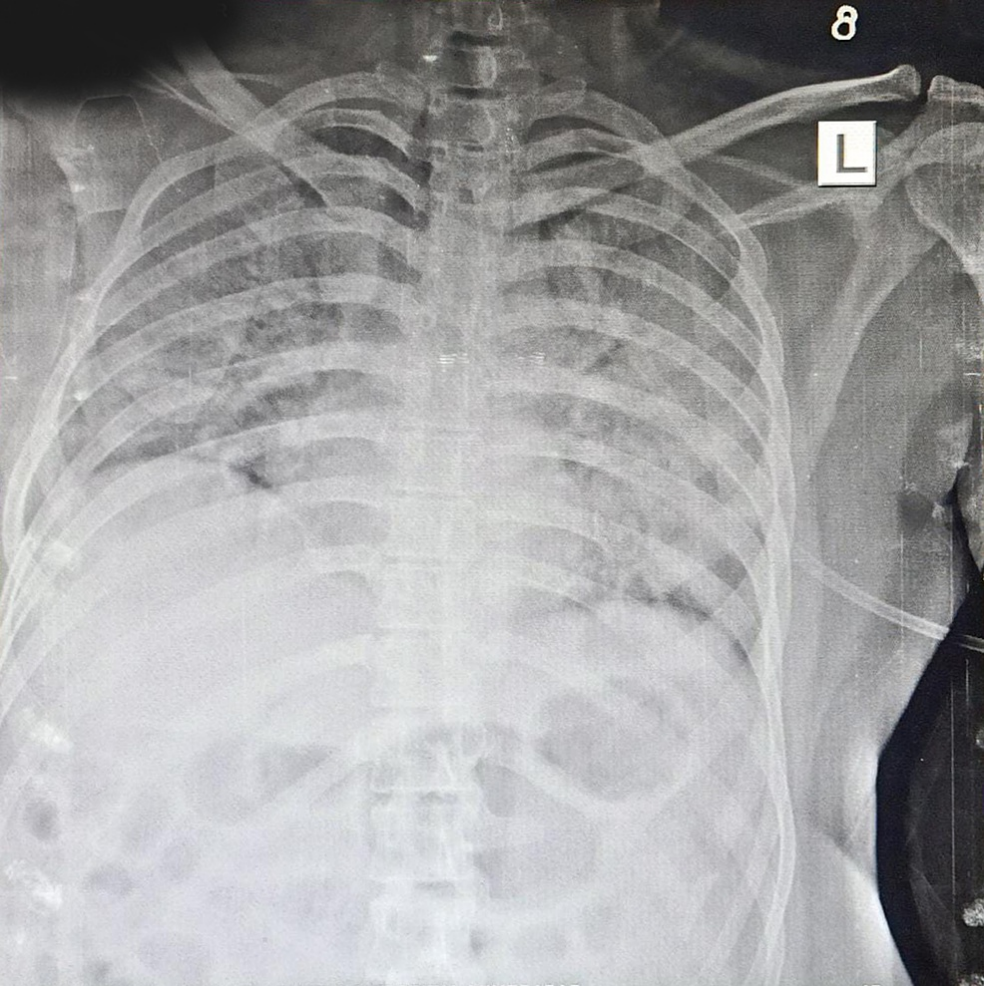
X-ray of case 2 before bevacizumab
